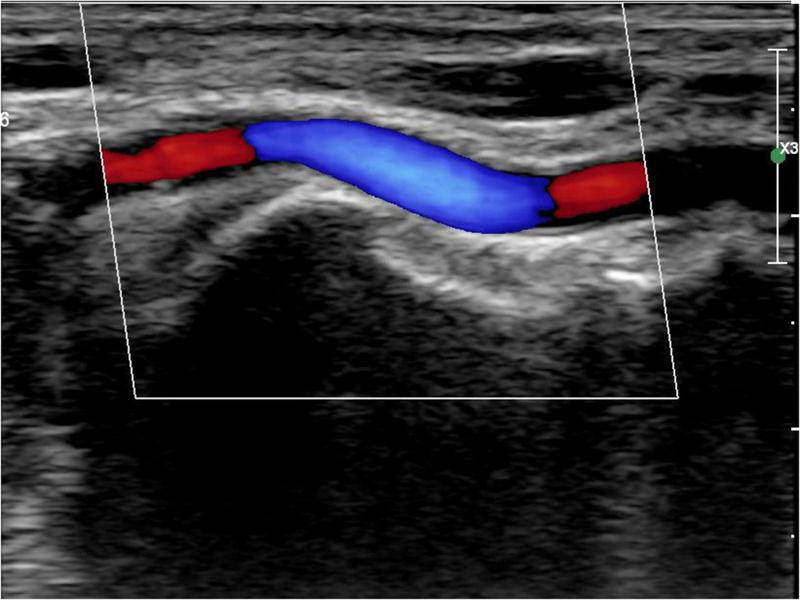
Distal radial artery ultrasound.
